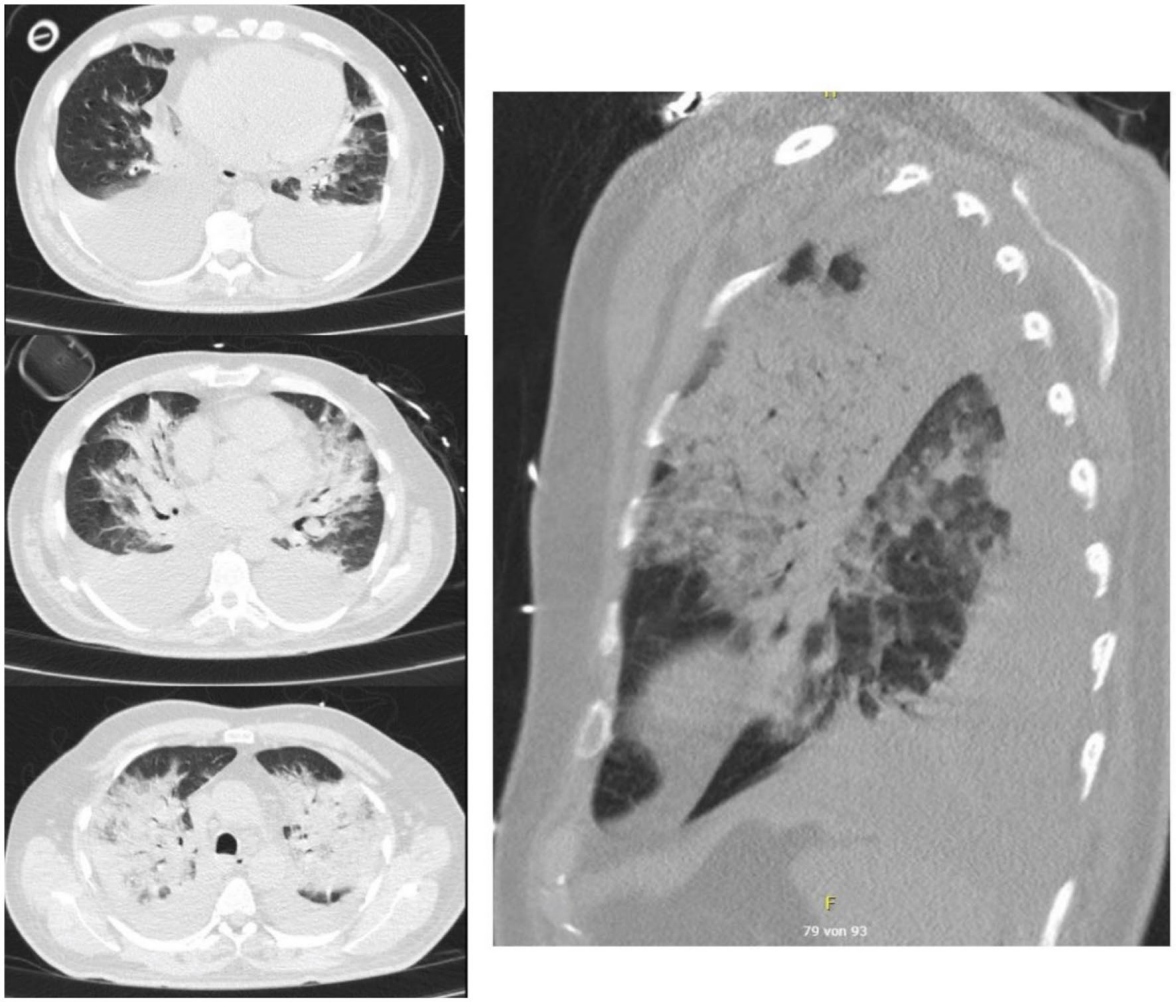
Non-contrasted thoracic CT-scan, postoperative day 7. 3 transversal views from supradiaphragmal to apical (left). 1 sagittal view (right). Progredient consolidations of both upper lobs. Progredient bilateral pleura effusion
